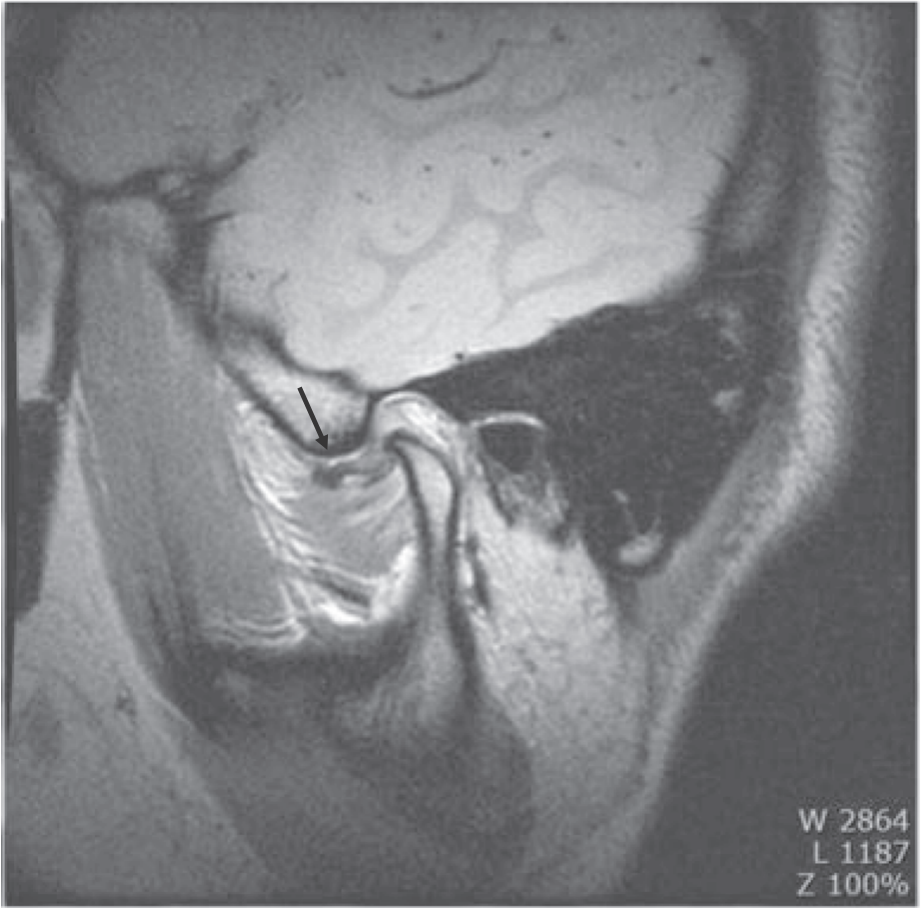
MRI shows the posterior band of the disc was anterior to the superior part of the condylar head. (Arrow indicates displaced disc anteriorly)
